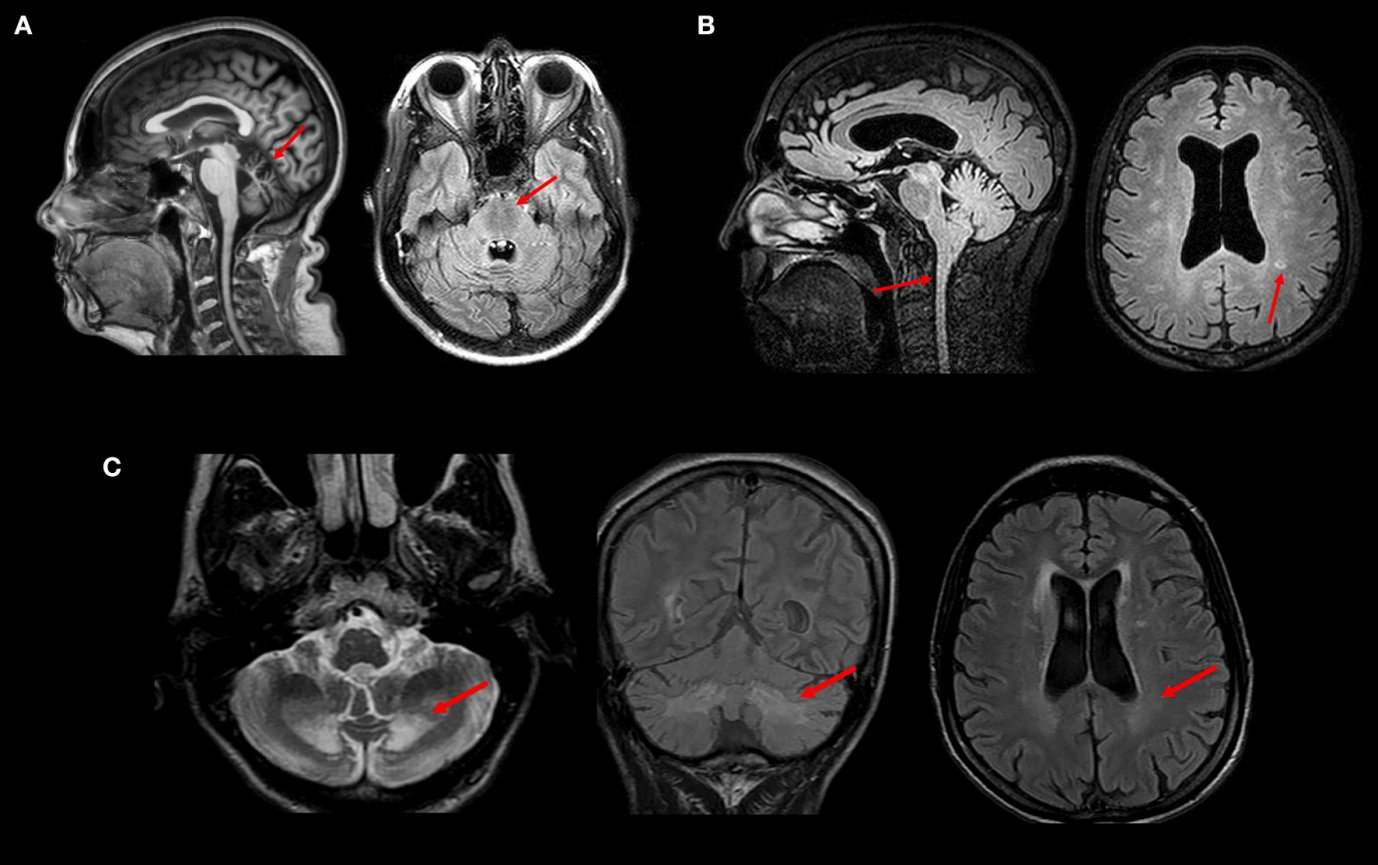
MRI and clinical findings in patients with inherited diseases that may mimic HSP—(A) Superior vermis atrophy (T1W image) and linear hypointensities (FLAIR image) in the pons in a patient with ARSACS. (B) Spinal cord atrophy and white matter hyperintensities (FLAIR images) in a patient with Adrenomyeloneuropathy. (C) Peridentate and periventricular white matter signal changes (FLAIR and T2W images) in a patient with Cerebrotendinous Xanthomatosis. All images were obtained during routine clinical care of these patients at UNICAMP and UNIFESP hospitals.
